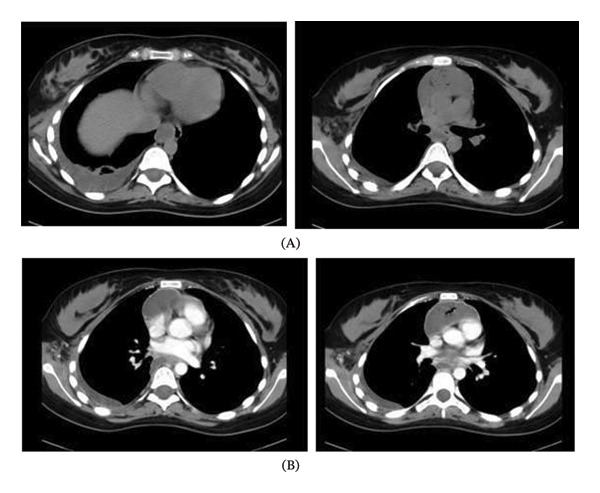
(A) The 2nd computed tomography (CT) scan at 11.01.2020. (B) The 3rd computed tomography (CT) SCAN on 11.2.2020.
